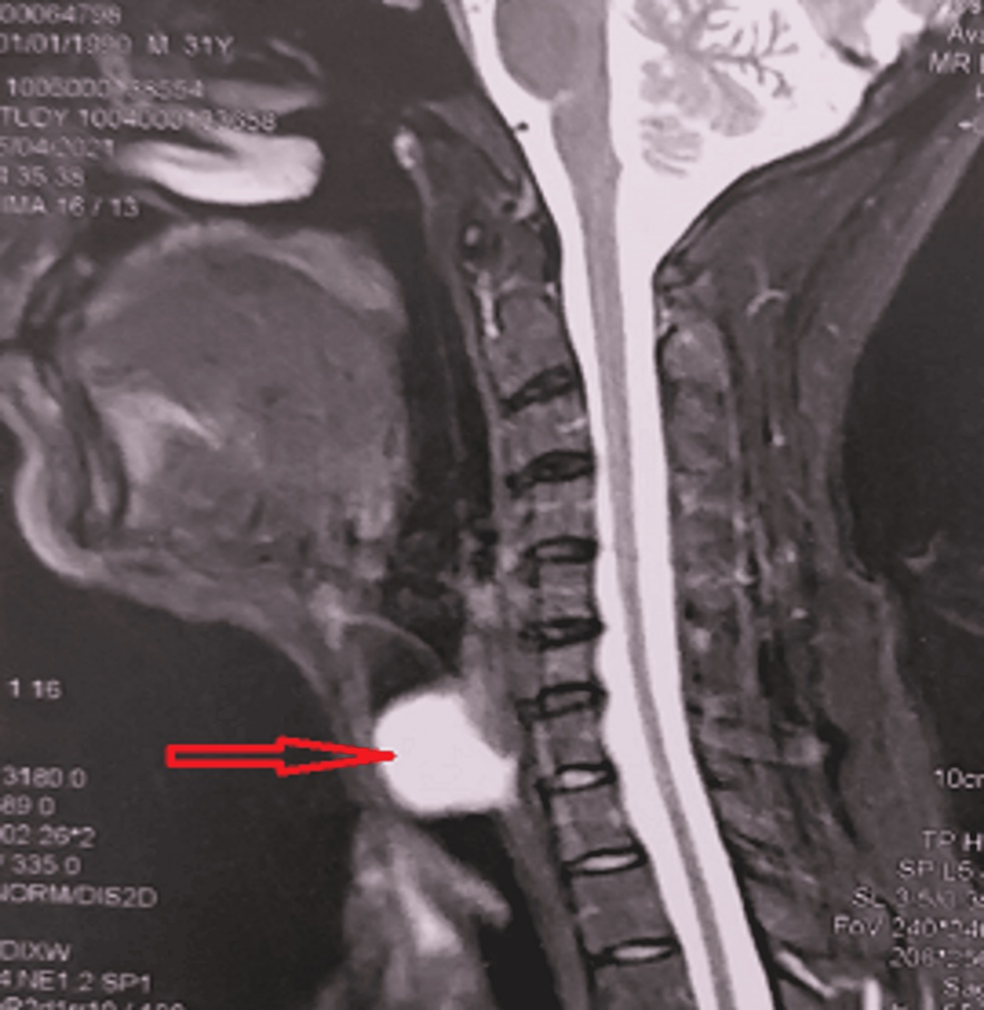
Sagittal T2 MRI showing the intra-tracheal pathologic process.
